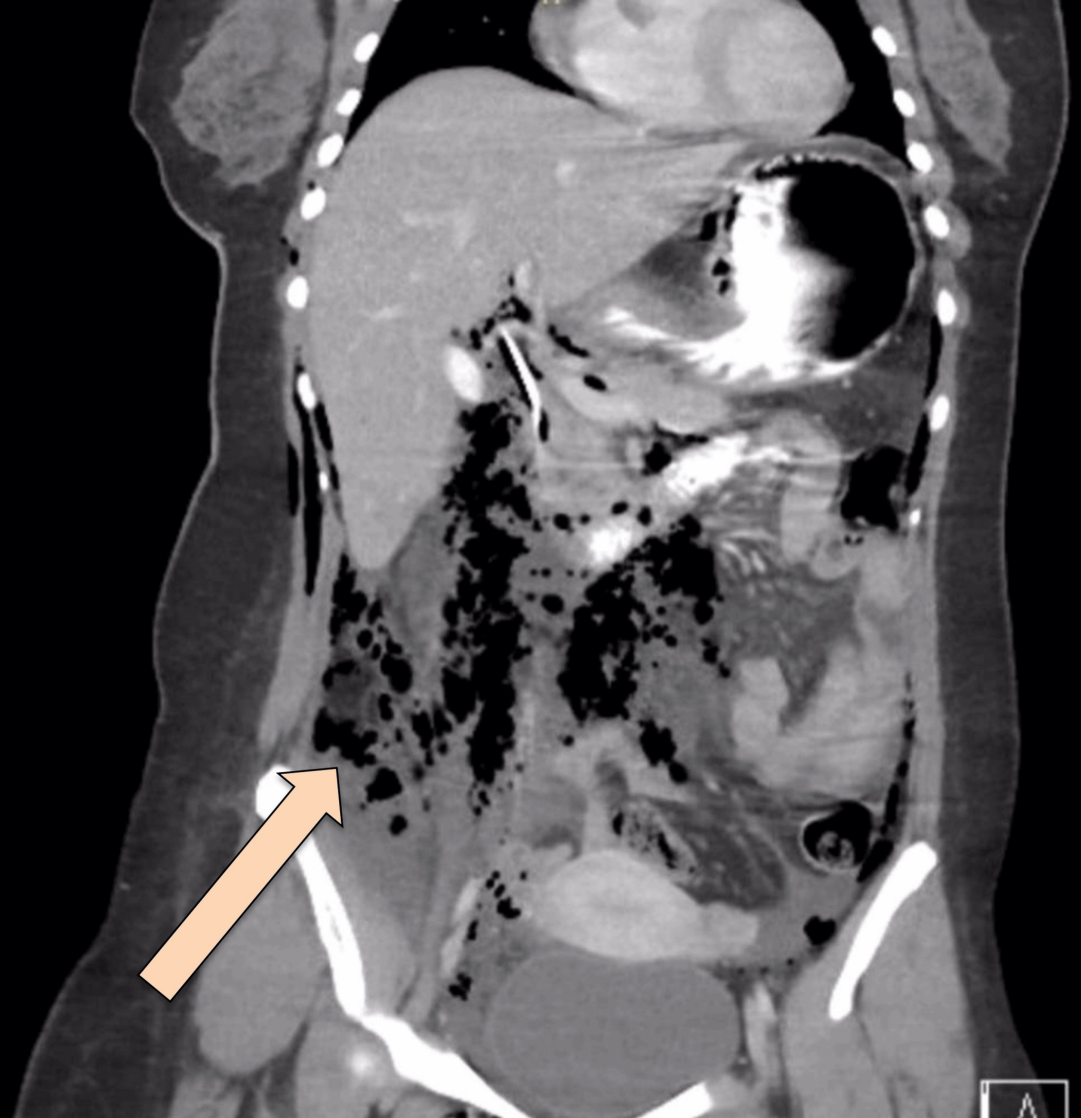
Coronal view of CT abdomen with IV and oral contrast post-ERCP showing extensive pneumoperitoneum The arrow is pointing at the extensive pneumoperitoneum CT: computed tomography; IV: intravenous; ERCP: endoscopic retrograde cholangiopancreatography
